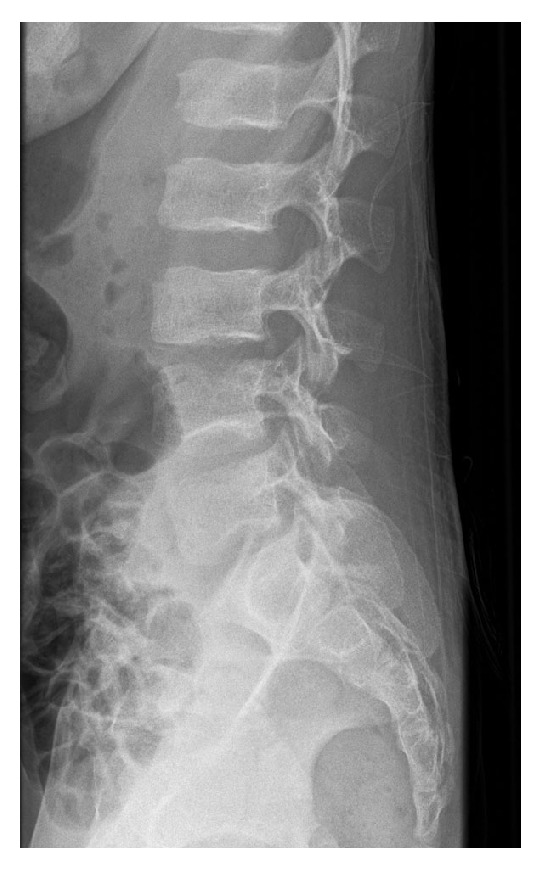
Lateral radiograph of the lumbar spine 4 days after the surgery shows L3-L4 disk space narrowing with erosion of the inferior endplate of L3 and superior endplate of L4.
